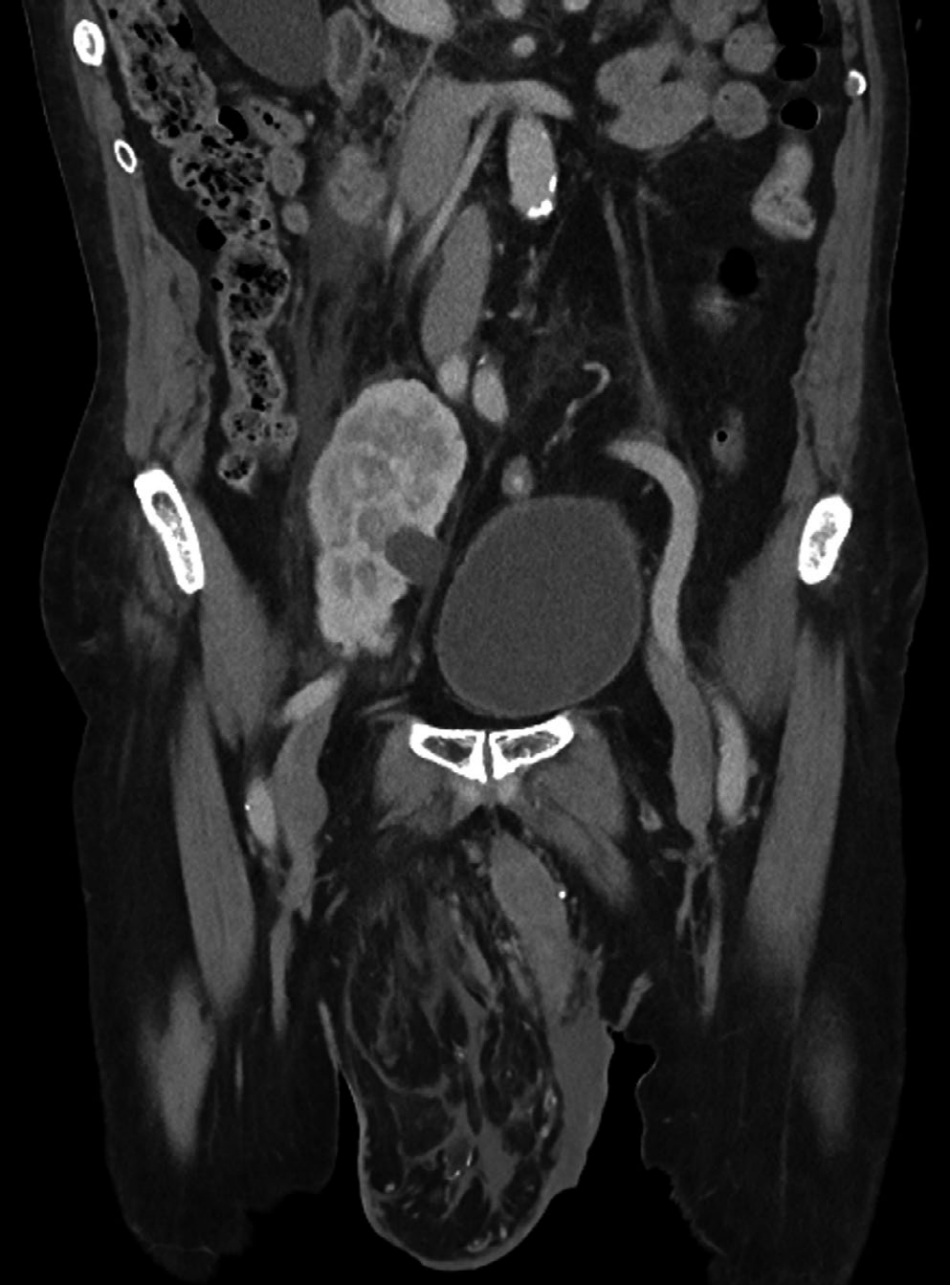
A 78‐y‐old gentleman was seen in the vascular surgery clinic due to peripheral arterial disease. Computed tomography discovered unconventional visceral arrangement. In this case, a large right inguinal hernia has distorted the usual retroperitoneal anatomy and migrated the right kidney to the right iliac fossa, approaching the hernia neck. The right kidney exhibits a degree of hydronephrosis
